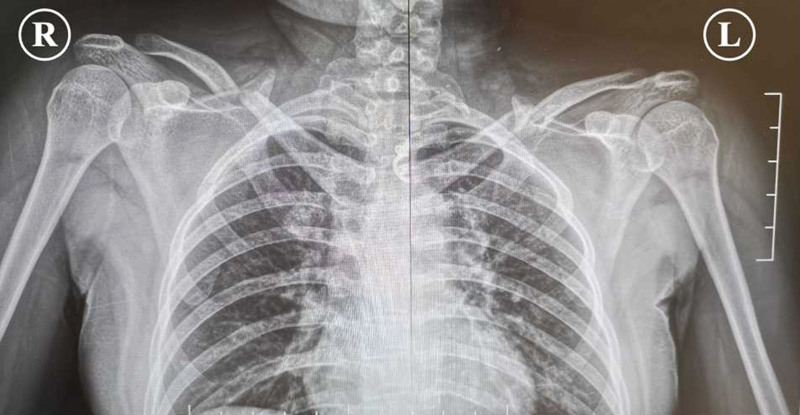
X-ray images on initial presentation: bilateral fractures of the middle clavicle.
